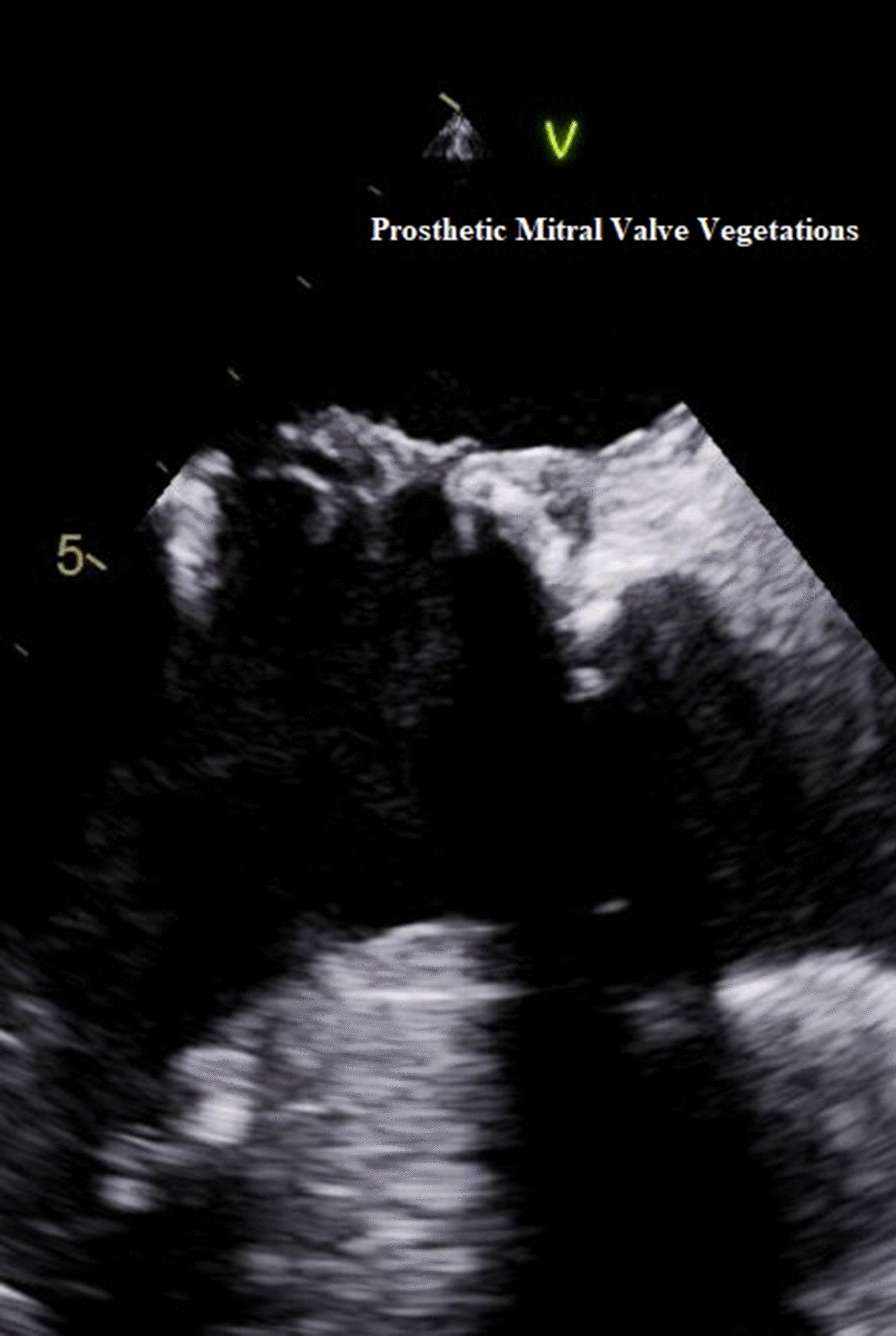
Transesophageal echocardiography showing the prosthetic mitral valve with vegetations attached to the atrial surface
